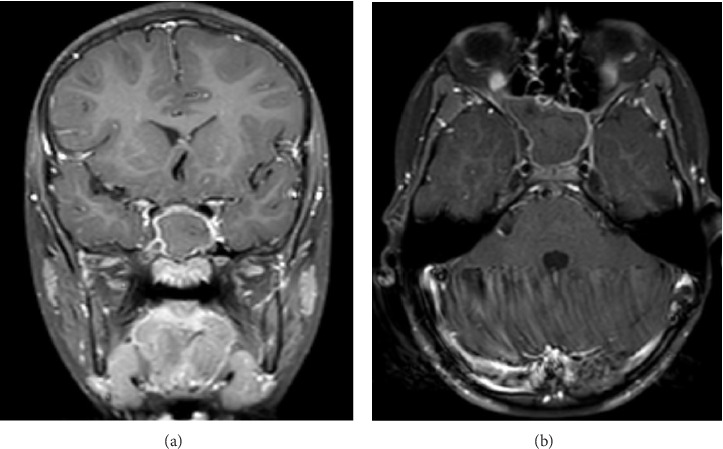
Brain MRI showing sphenoid lesion on T1 sequence with IV gadolinium: coronal view (a); axial view (b).
